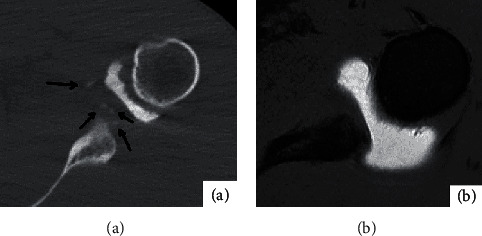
Male, 27 years old, anterior dislocation of left shoulder for 3 months. (a) shows the transverse bone window image of CTA on the left shoulder. Black arrow shows that several high-density bone fragments can be seen beside the lower margin of the glenoid cavity. (b) shows the T1WI-FS image in the transverse axis of the left shoulder MRA, and no obvious bone fragments are seen beside the lower margin of the glenoid cavity.
